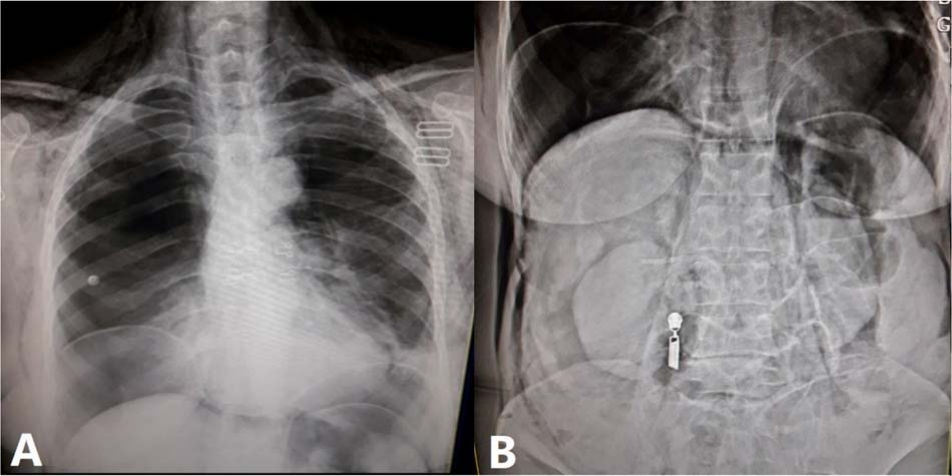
Chest X-ray. (A) Represents a chest X-ray revealing the presence of right pneumothorax with right lung atelectasis, pneumopericardium, and pneumoretroperitoneum. (B) Abdominal X-ray demonstrating the presence of pneumoperitoneum and pneumoretroperitoneum.
